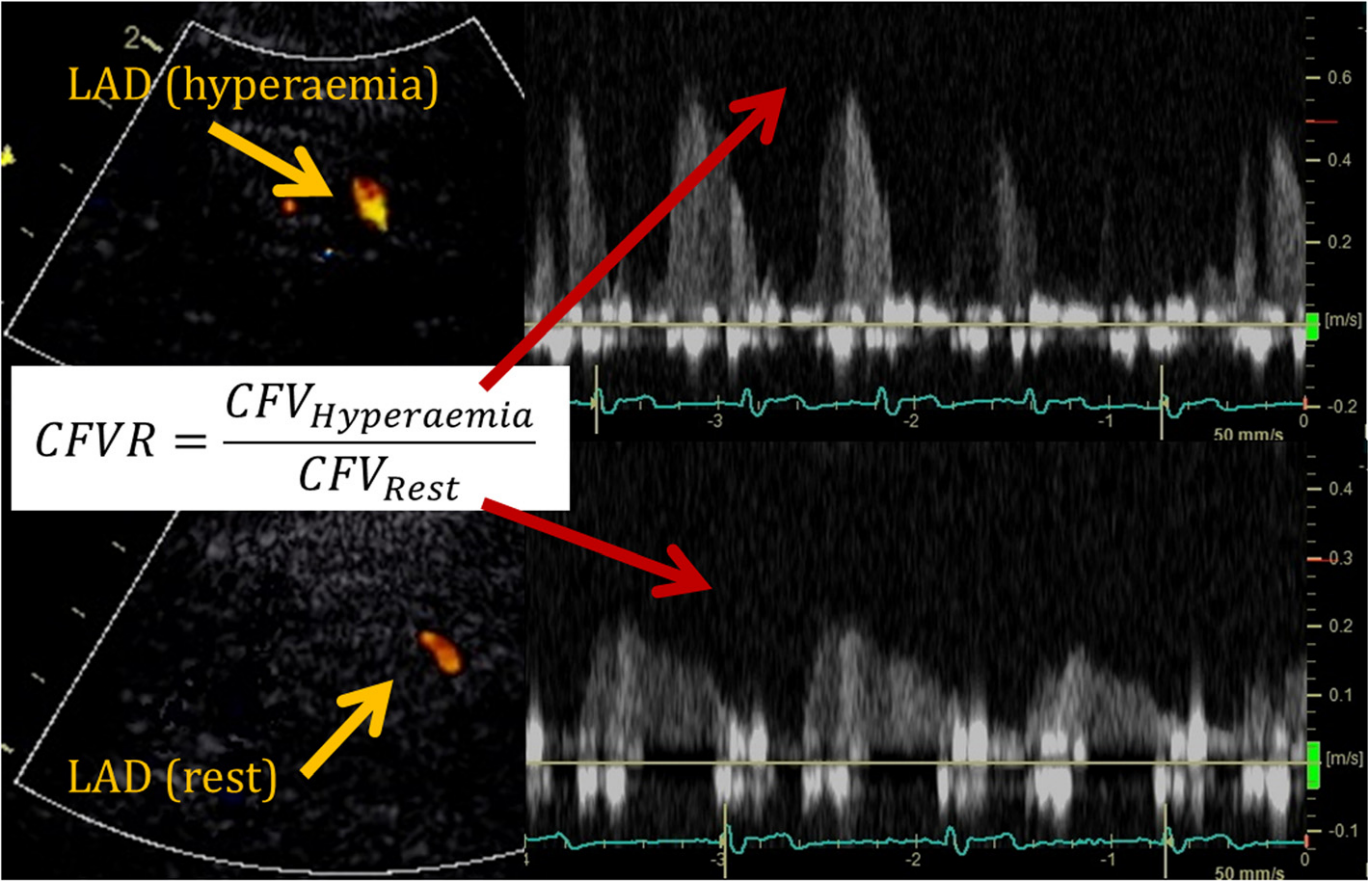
Example of CFVR measurement by Doppler TTE. Lower pictures show measurement at rest. Upper pictures show measurement during hyperaemia. Pictures to the left show the colour Doppler visualisation of the LAD. Pictures to the right show the pulsed wave Doppler measurement of the diastolic peak coronary flow velocity (CFV). Coronary flow velocity reserve (CFVR) of this participant was 2.86 (corresponding MFR_LAD_ was 2.57)
